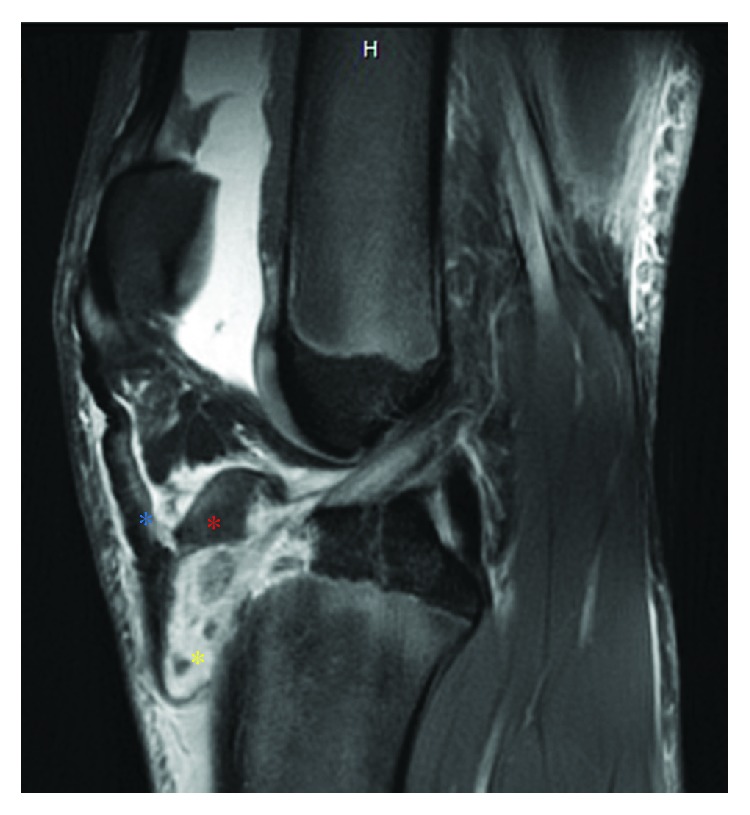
Preoperative sagittal view of MRI demonstrating tibial tubercle avulsion fracture (red asterisk), distal patellar tendon rupture (blue asterisk), and hematoma formation (yellow asterisk) at the site of injury.
